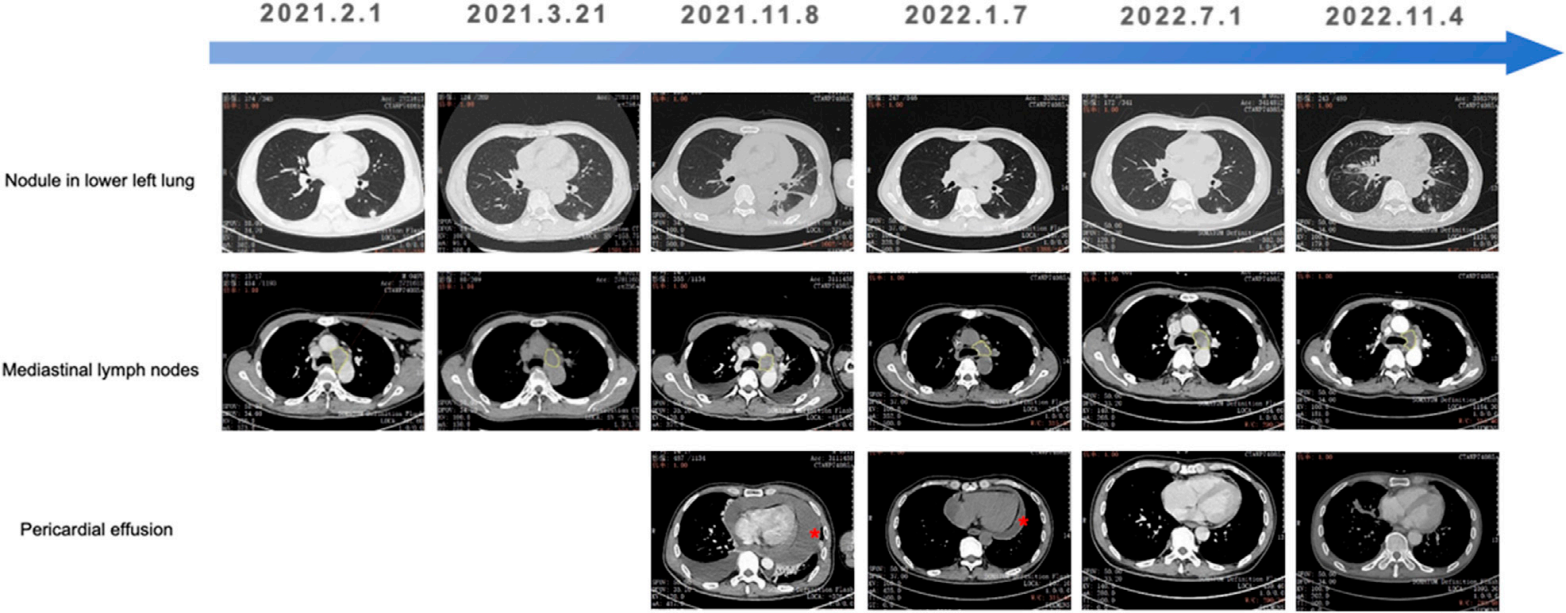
Longitudinal CT imaging of the patient’s thoracic lesions.
